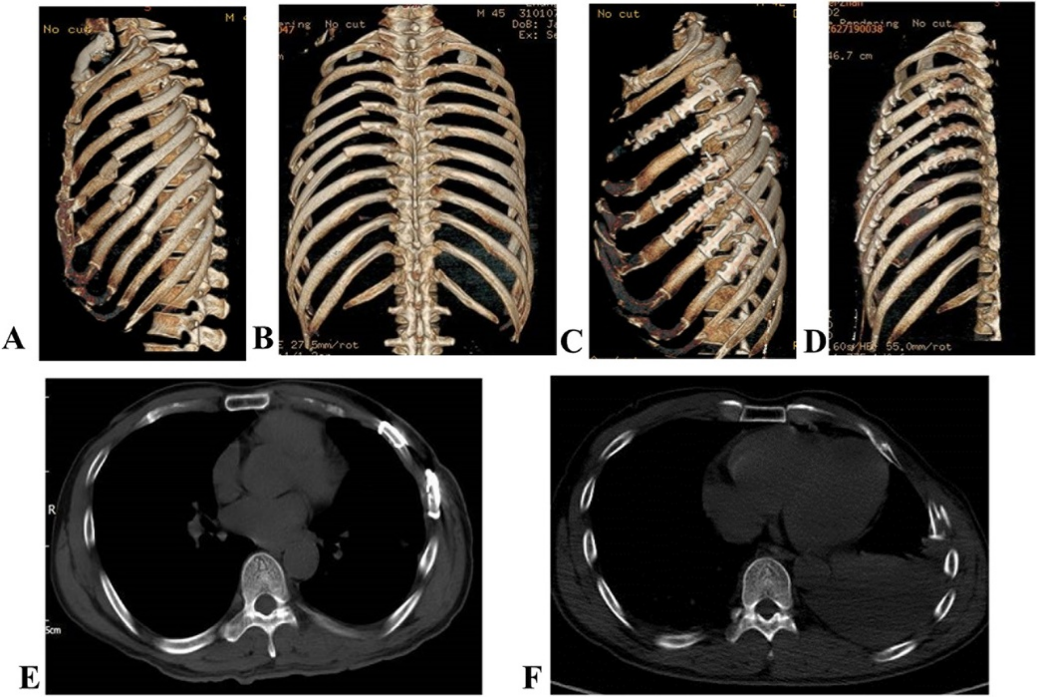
One typical case of rib fracture before and after surgical fixation. a-b, a 3- dimensional reconstruction CT image showing fractured rib before surgery. (c-d), a 3-dimensional reconstruction CT image after the surgery. (e), a chest CT image showing fractured rib before the surgery. (f), a chest CT image showing fractured rib after the surgery. Collapse of the thorax is corrected after the surgical fixation
